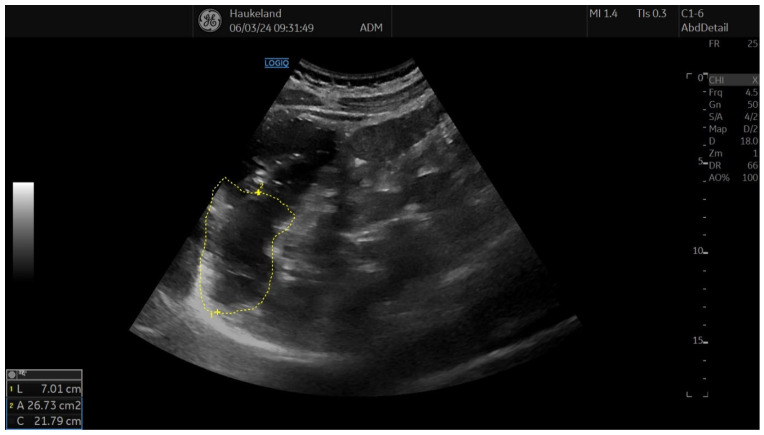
This image shows a measurement of proximal gastric area (PGA) using 2D-US in sagittal section, and this is used to assess gastric accommodation. PGA was obtained by placing the transducer longitudinally under the left subcostal margin and tilting it cranially in the long axial direction. Internal landmarks to look for were the left kidney in the longitudinal projection, the left lobe of the liver, and the tail of the pancreas. PGA was calculated by tracing along the luminal echogenic surface, which corresponded to the interface between the gastric liquid and the mucosa of the gastric wall. This was performed from the top margin of the fundus to 7 cm inferiorly along the axis of the proximal stomach. This image was taken after meat soup ingestion as part of the ultrasound meal accommodation test (UMAT) that is regularly performed at Haukeland University Hospital, Bergen, Norway.
